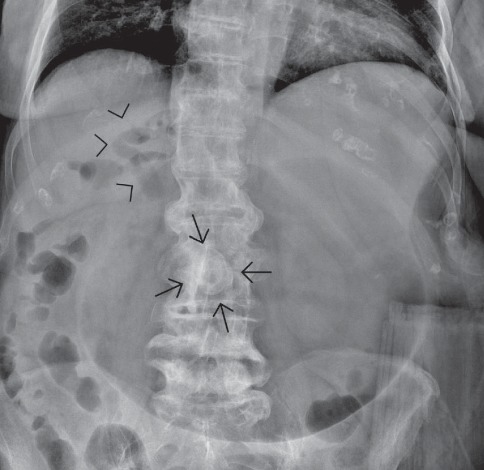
Rigler's triad in a supine abdominal radiograph. Note is made of the association of pneumobilia (arrowheads), a peripherally calcified stone (arrows) superimposed to the lumbar spine, and evident gastric overdistension; findings are consistent with gastric outlet obstruction due to a migrated large gallstone.
